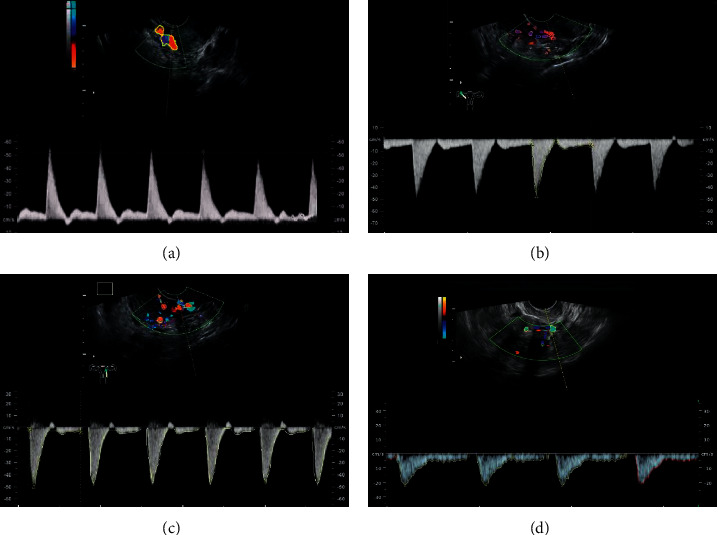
Ultrasound image during pregnancy. (a) Normal uterine artery ultrasound of normal pregnant women; (b) abnormal uterine artery ultrasound of normal pregnant women; (c) abnormal uterine artery under ultrasound based on STFT algorithm; (d) abnormal uterine artery under ultrasound based on EWT algorithm.
